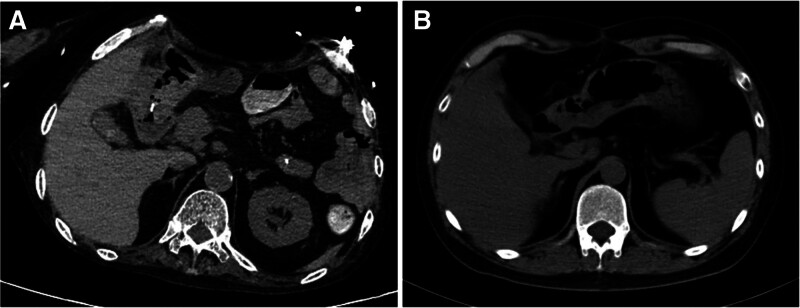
The reexamination CT scan shows clear fat tissue around the stomach, with no obvious signs of fluid accumulation or air accumulation. CT = computerized tomography.
